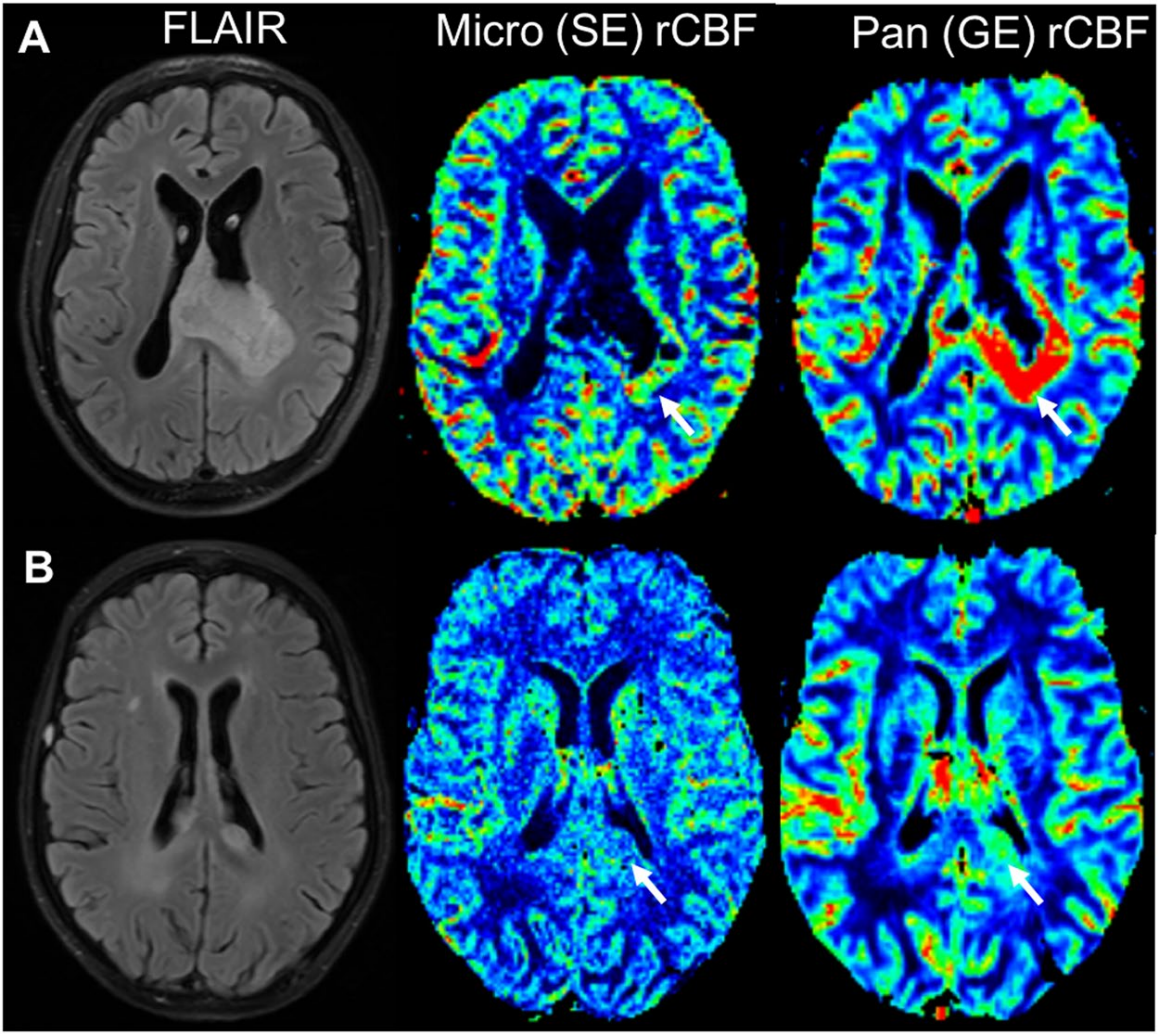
FLAIR and DSC-derived perfusion maps in a patient with below-median (A) and above-median (B) PFS and OS at baseline. Patient 9 (A) had a large FLAIR-hyperintense tumor involving the splenium of the corpus callosum with significantly elevated concomitant rCBF, especially on GE sequences (white arrows). PFS was 3.8 months and the patient died 8.7 months after diagnosis. The tumor in Patient 8 (B), on the other hand, only demonstrated minimally elevated rCBF at baseline. PFS and OS were 7.1 and 30.3 months, respectively.
